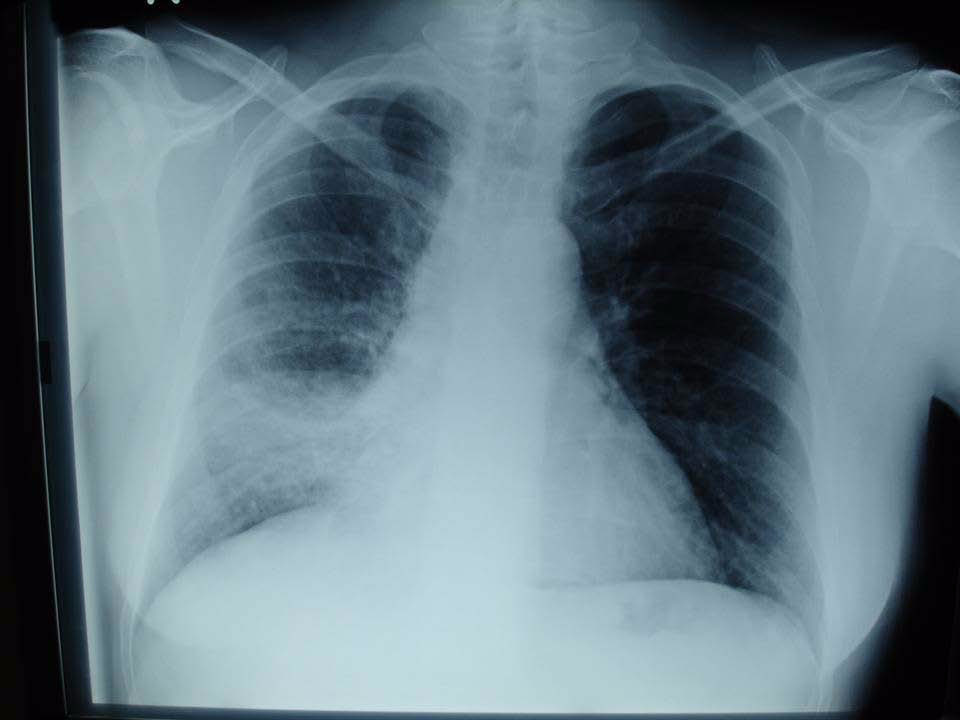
Chest radiography from a patient with right lower lobe pneumonia.
